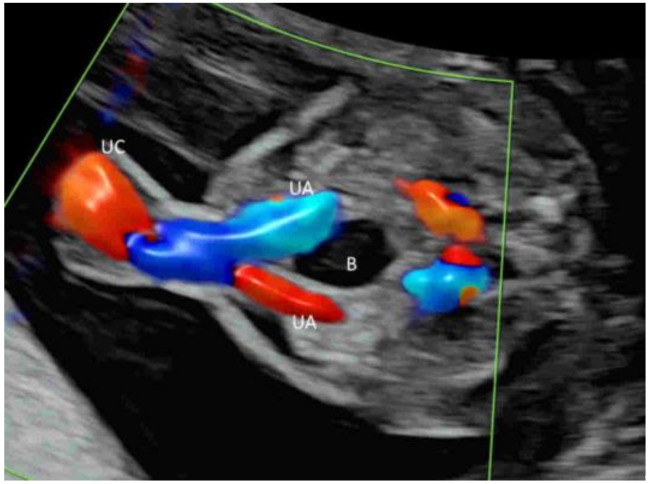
Color Doppler in a transverse plane of a normal fetus at 20 weeks’ gestation showing two umbilical arteries (UA) surrounding the urinary bladder (B). UC, umbilical cord.
